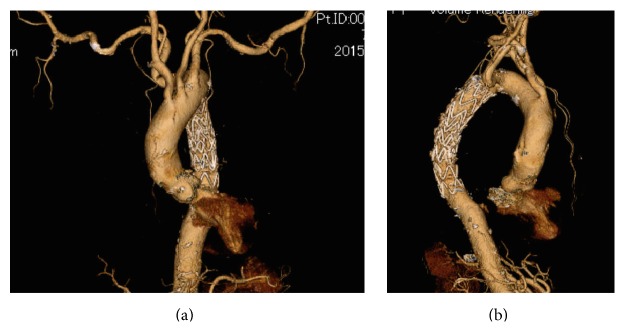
Three-dimensional CT findings at postoperative day 12. (a) Anterior view. (b) Right lateral view. The disappearance of ULP was confirmed by contrast-enhanced CT, and no endoleaks were observed. The stent grafts were placed distal to the origin of the right subclavian artery so the tips of the bare stents did not come into contact with the aortic wall.
